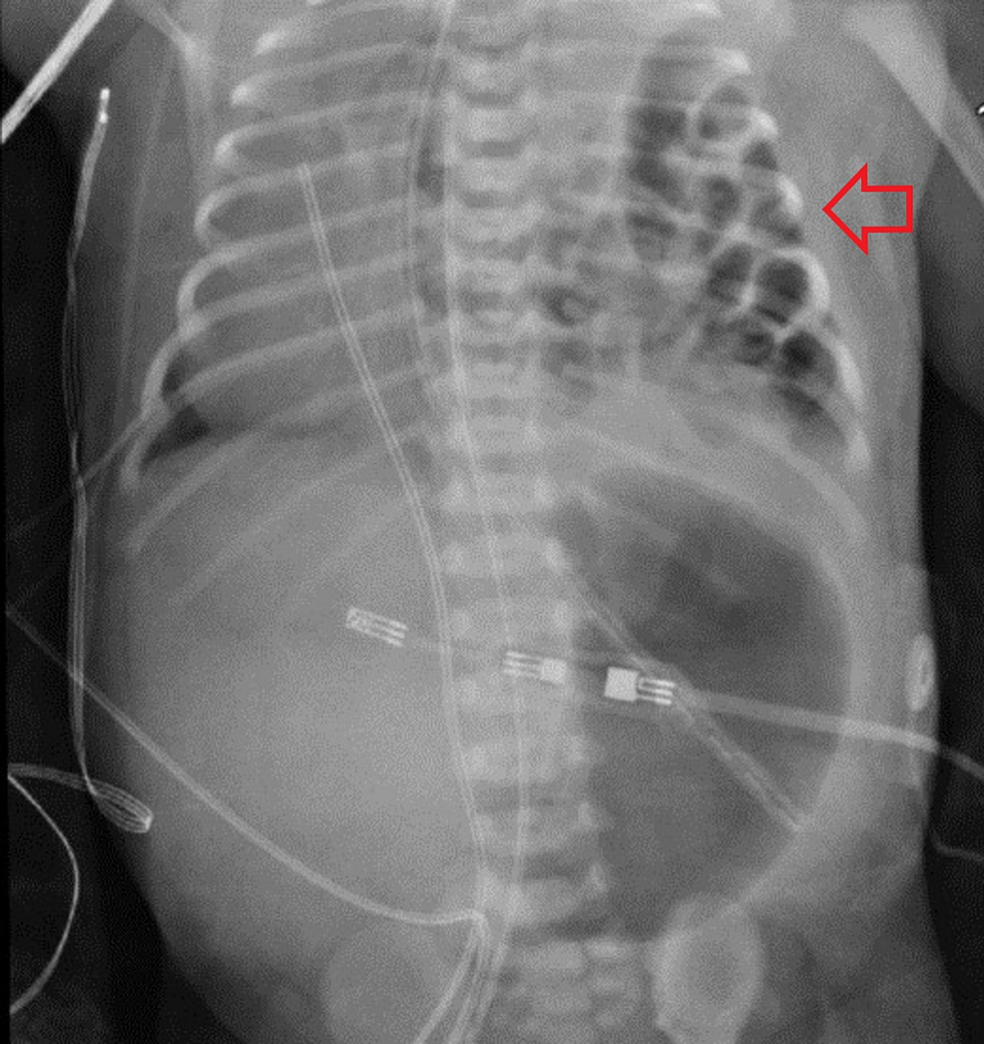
Chest X-ray of the congenital diaphragmatic hernia (arrow)
